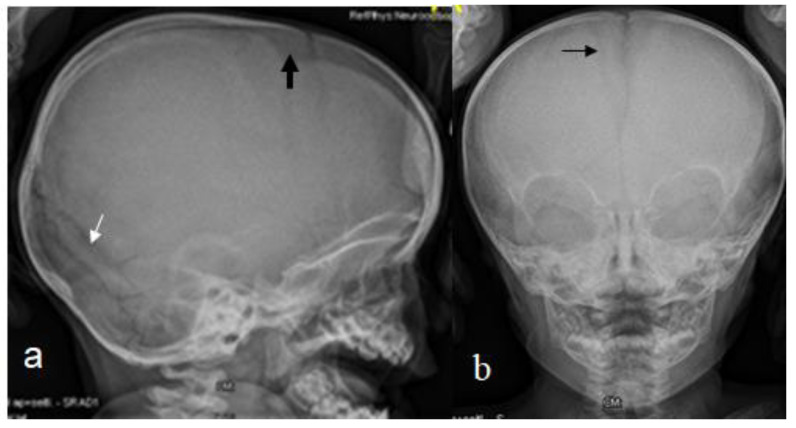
Lateral skull radiograph of a 14-month-old boy with RSS showed a very large anterior fontanelle (massive defective ossification) associated with frontal bossing, giving the impression of pseudo-hydrocephalus-arrow hyperostosis of the skull base and Wormian bones along the lambdoid sutures (a). AP skull radiograph showed the frontal bossing and the persistence of the anterior fontanelle-arrow (b).
